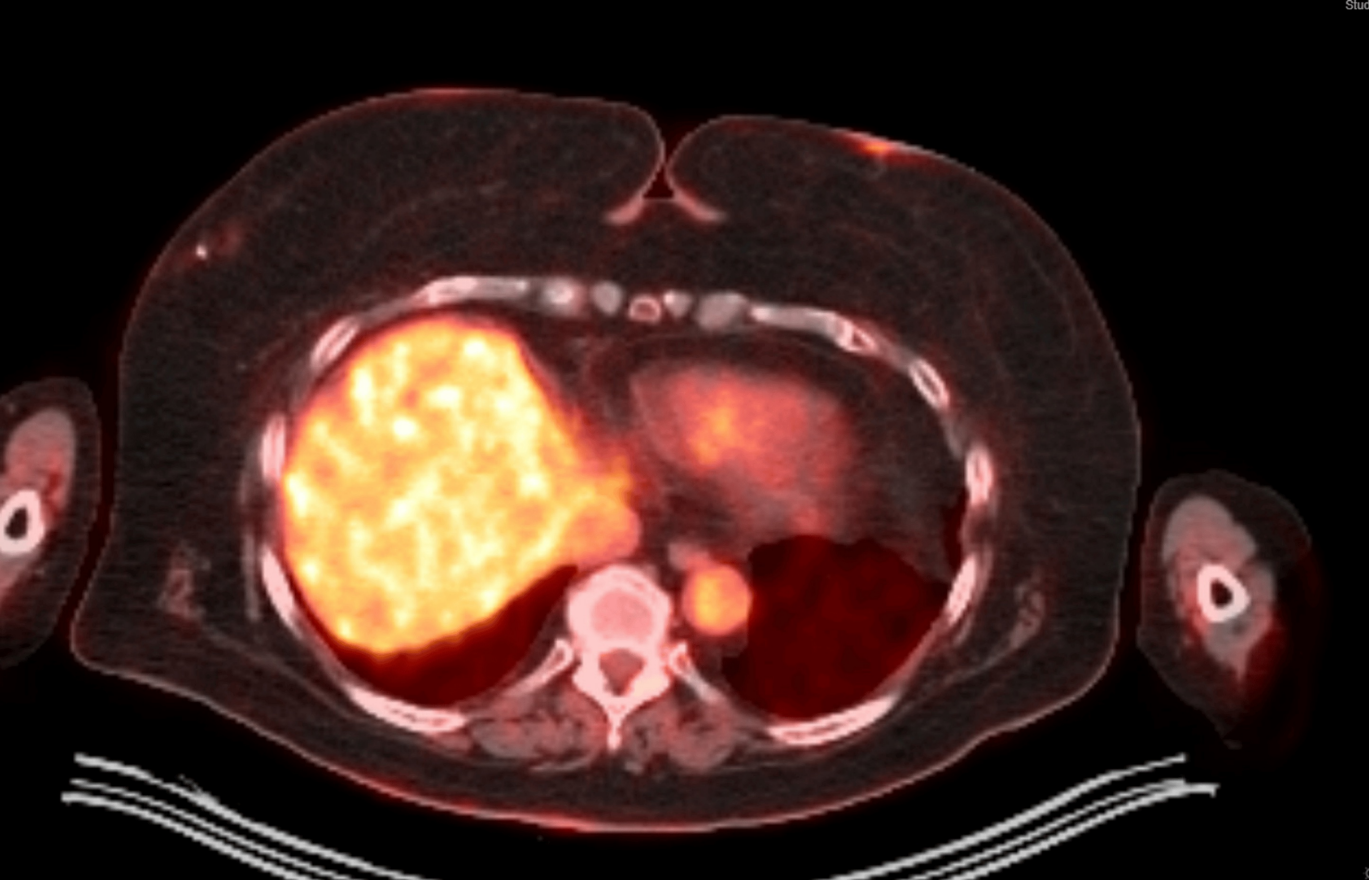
PET-CT (axial view) showing slightly more inferior view compared to Figure 1. PET showing an avid lesion at the 10 o'clock position in the right breast that was biopsied, with a biopsy clip in place. This was biopsy-proven to be invasive lobular carcinoma of the breast.
